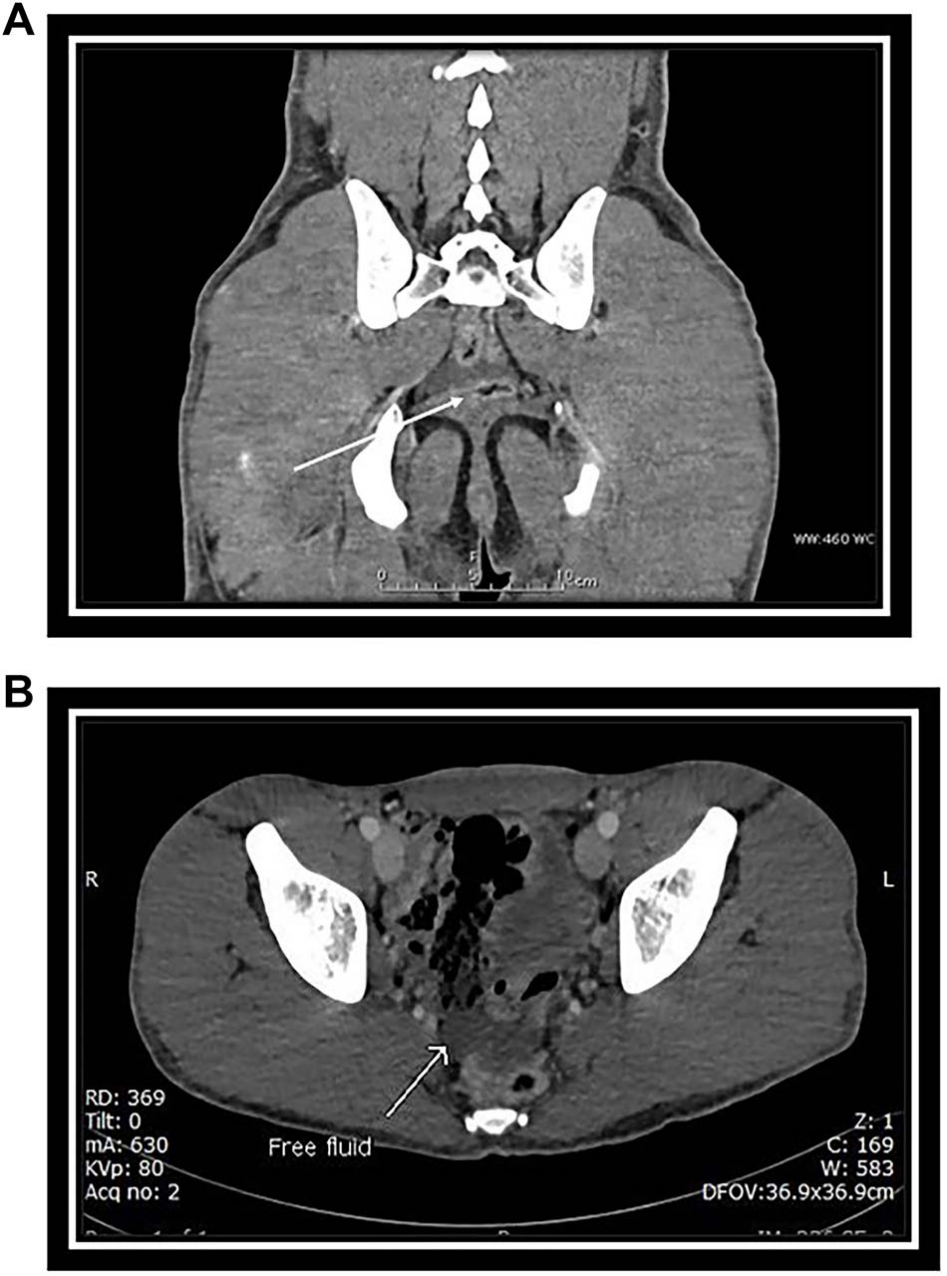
(A) Coronal CT scan section: arrow indicates free fluid in the Douglas pouch. (B) Axial CT scan section: arrow indicates free fluid in the Douglas pouch.
